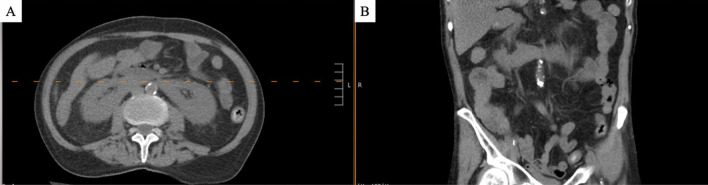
Horseshoe kidney in A transverse and B coronal view of CT abdomen
